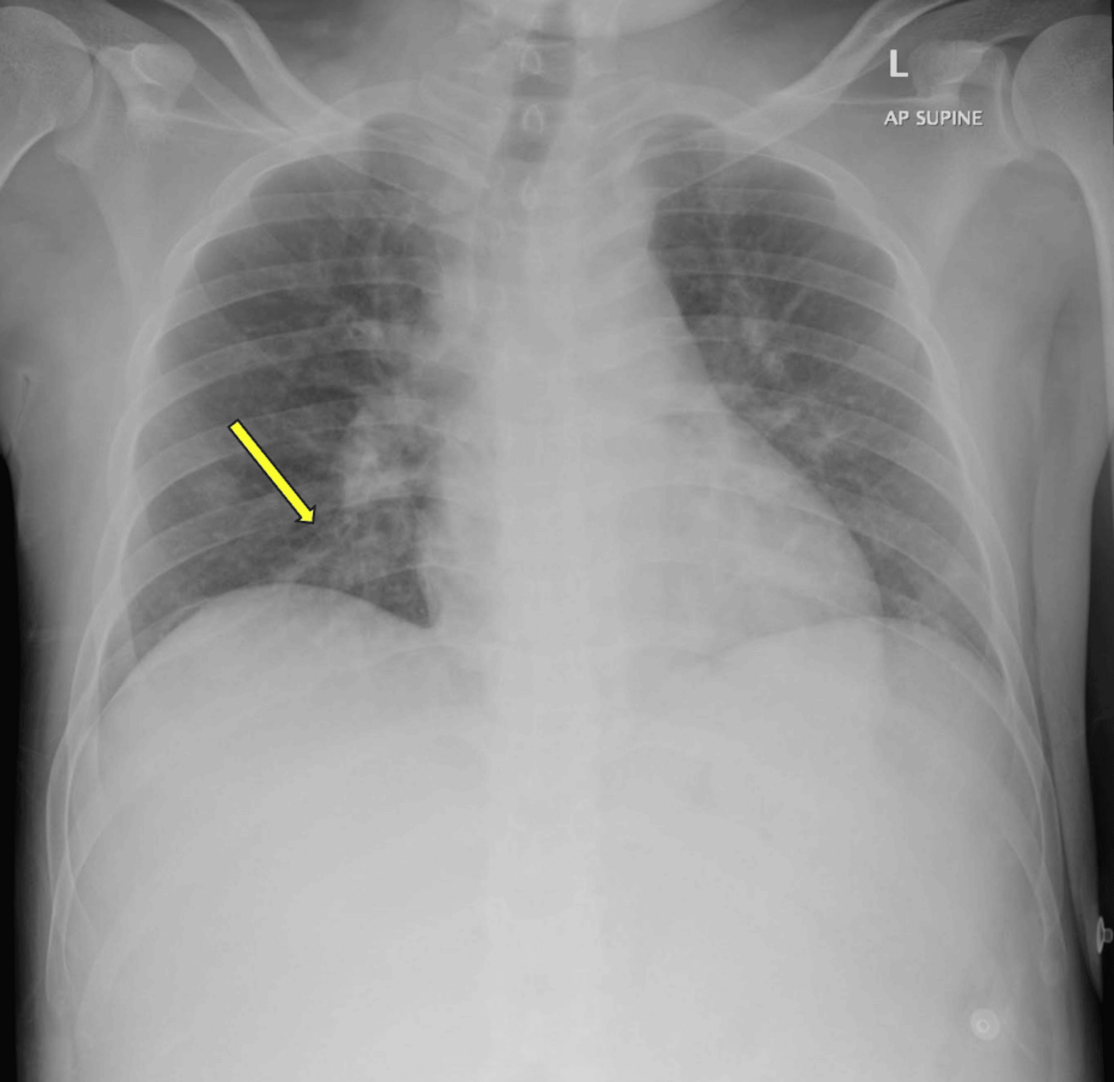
Chest X-ray performed on presentation showing poorly defined infiltrates in the right lower lung zone (yellow arrow) A follow-up chest X-ray was performed on the 24th day of admission and showed no significant changes.
